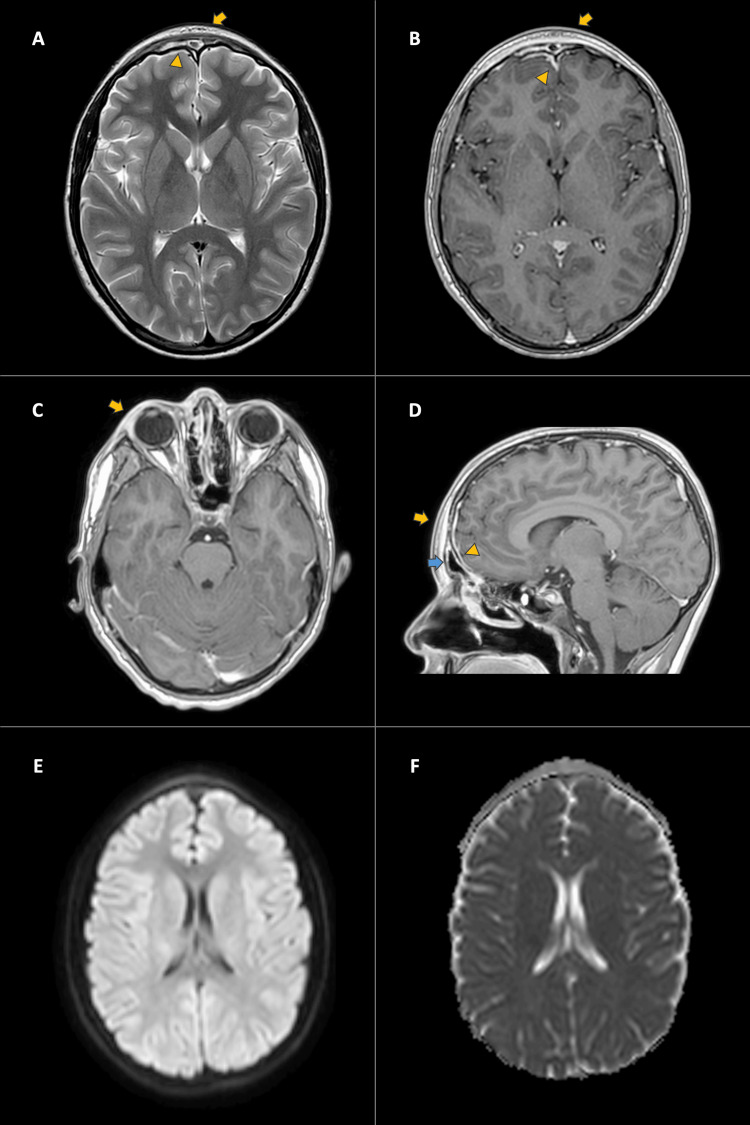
Contrast-enhanced MRI of the skull (control). A. T2 - Axial: Resolution of inflammatory changes and abscess in the frontal soft tissues (Arrow). Residual thickening and enhancement of the frontal sinus (Arrowhead). B. Contrast-enhanced T1 - Axial: Resolution of inflammatory changes and collection in the frontal soft tissues (Arrow). Residual leptomeningeal enhancement (Arrowhead). Resolution of frontal epidural collection. C. Contrast-enhanced T1 - Axial: Significant reduction in edema and enhancement of the right periorbital soft tissues (Arrow). D. Contrast-enhanced T1 - Sagittal: Resolution of inflammatory changes and collection in the soft tissues of the forehead (Yellow Arrow). Residual thickening and enhancement of the frontal sinus (Blue Arrow). Residual leptomeningeal enhancement (Arrowhead). E-F. DWI and ADC Map: No areas of restricted diffusion due to the resolution of epidural and frontal soft tissue abscesses. T2: T2-weighted MRI; T1: T1-weighted MRI; DWI: Diffusion-weighted imaging; ADC: Apparent diffusion coefficient.
